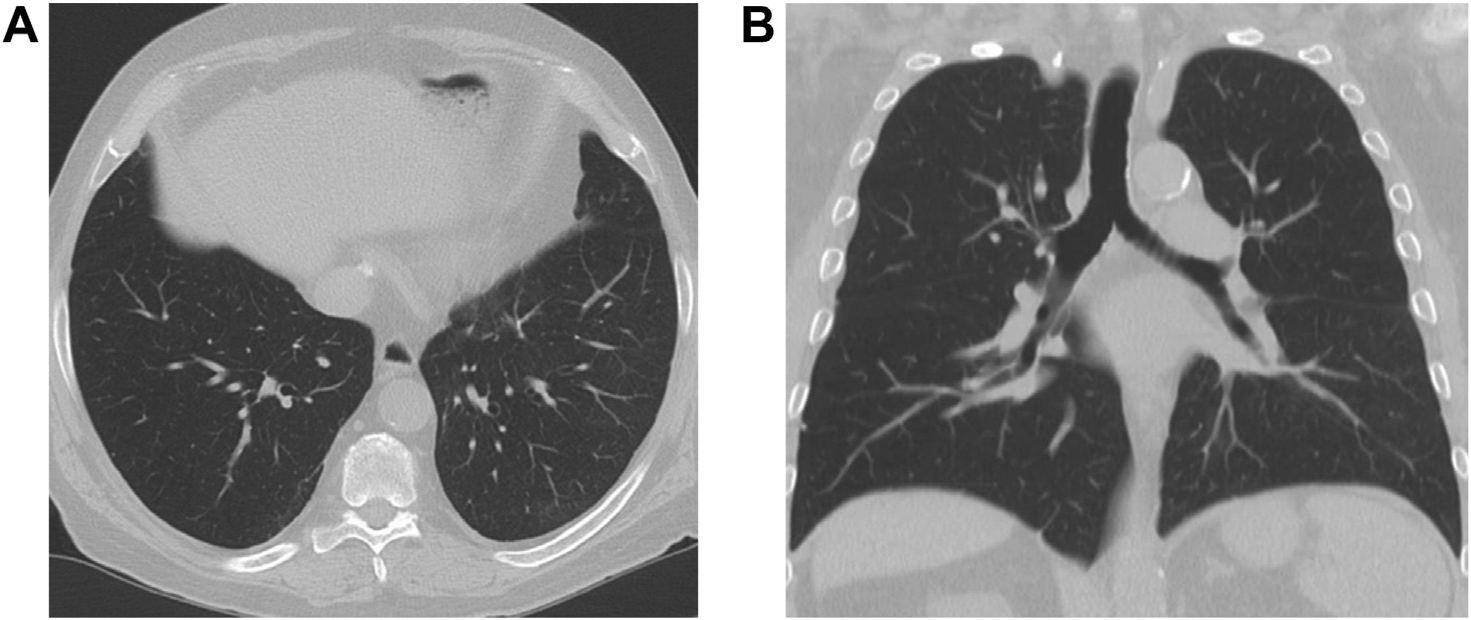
The final CT scan performed on the eight day of hospitalization showed only subtle residual ground glass opacities (GGO) in the left lower lobe (Figure 3A, transverse plane). Despite the GGO, no remaining structural lung changes in the context of pulmonary coronavirus disease 2019 (COVID-19) were present (Figure 3B, coronal plane).
